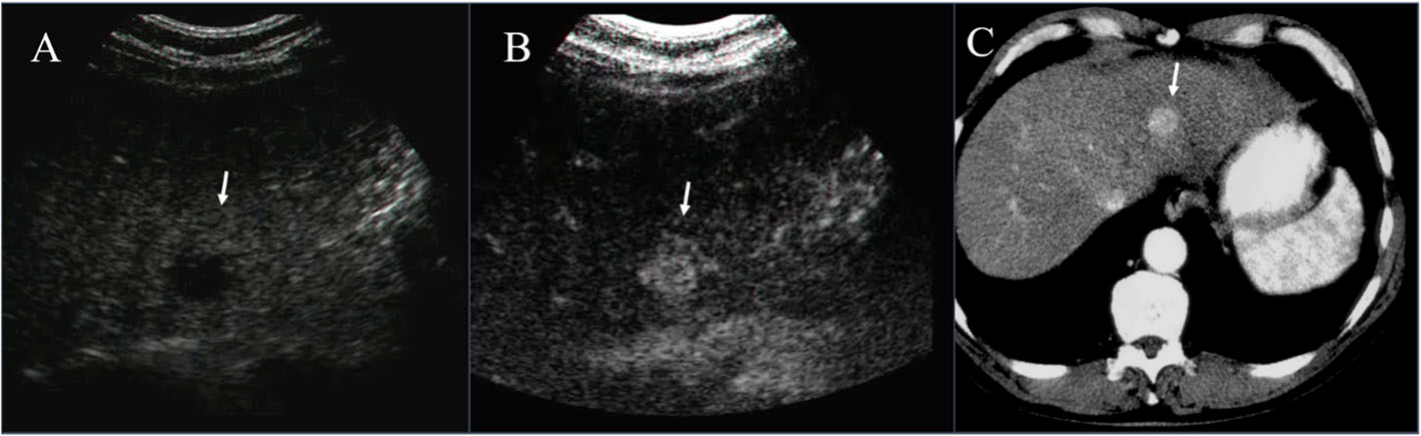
US (A), CEUS (B) and CT (C) assessment of HCC on II hepatic segment. The lesion in B and C (arrow) shows hyperenhancement during arterial phase of contrast study
